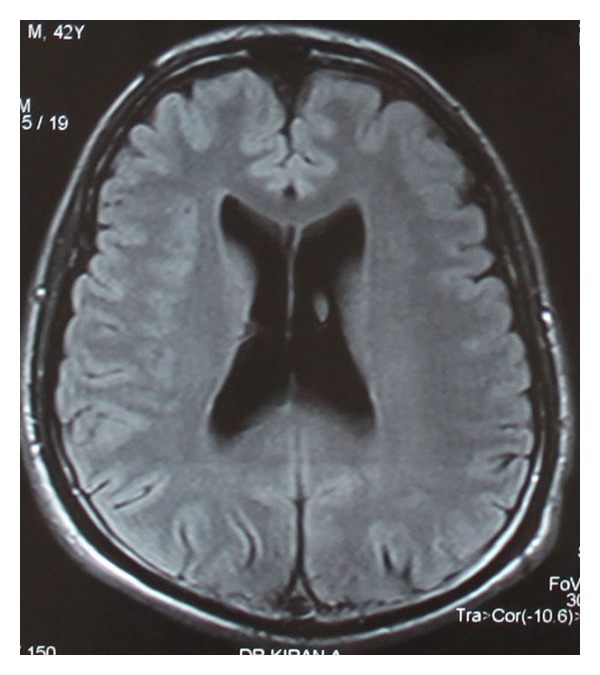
Pre-ECT cranial magnetic resonance imaging with contrast done to rule out organic causes of depression showing normal study.
